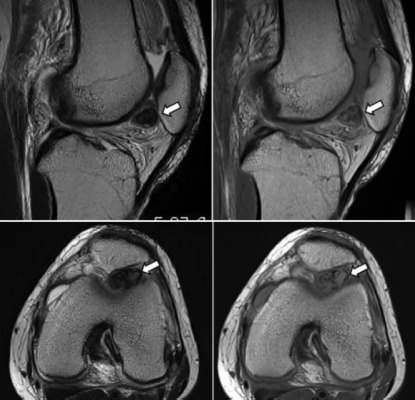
The MRI revealed a solitary mass in the knee joint cavity or infrapatellar fat pad that was hypointense-to-isointense in proton-density-weighted images and isointence-to-hyper-intense in T2-weighted images.
